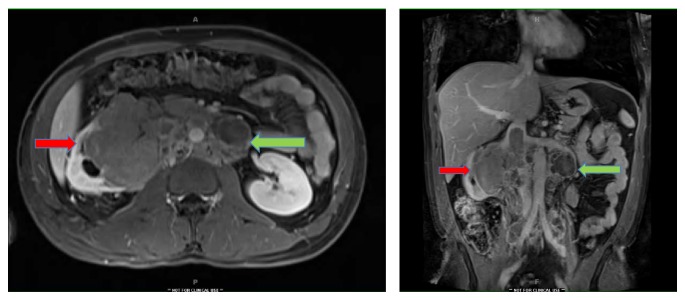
MRI showing right renal mass with IVC thrombus (red arrows) and retroperitoneal lymphadenopathy (green arrows).
